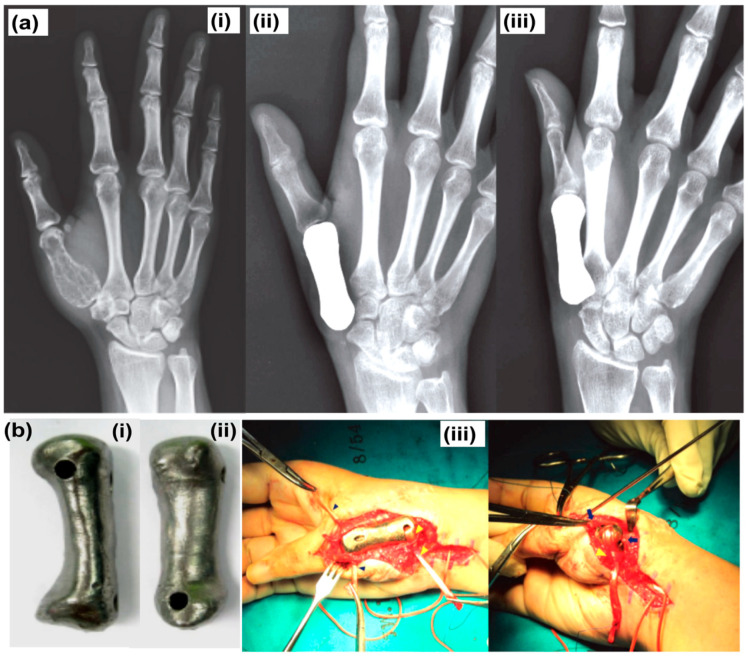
(a) (i) Radiograph demonstrates an expansile osteolytic lesion extending the first metacarpal’s full length. (ii,iii) Patient-matched first metacarpal prosthesis radiographs. (b) Before-implant-prosthesis images: (i) anterior aspect (ii) and volar aspect (iii). Intraoperative images showing 3D-printed titanium first metacarpal prosthesis with ligament reconstruction in proximal and distal portions: free palmaris longus tendon graft (blue arrow), flexor carpi radialis tendon (yellow arrow), and extensor pollicis brevis tendon (blue arrow). Reprinted with permission [175].
